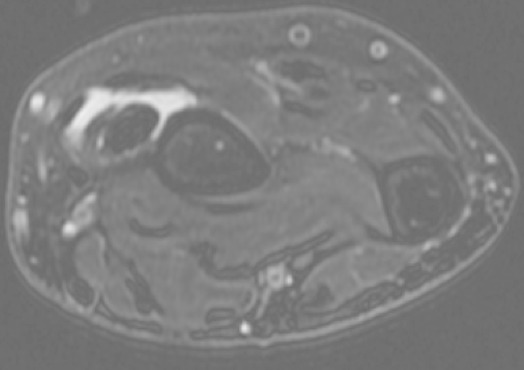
MRI axial view showing peritendinous effusion at the intersection of extensor carpi radialis brevis (ECRB), and extensor carpi radialis longus (ECRL) with extensor pollicis longus (EPL)
